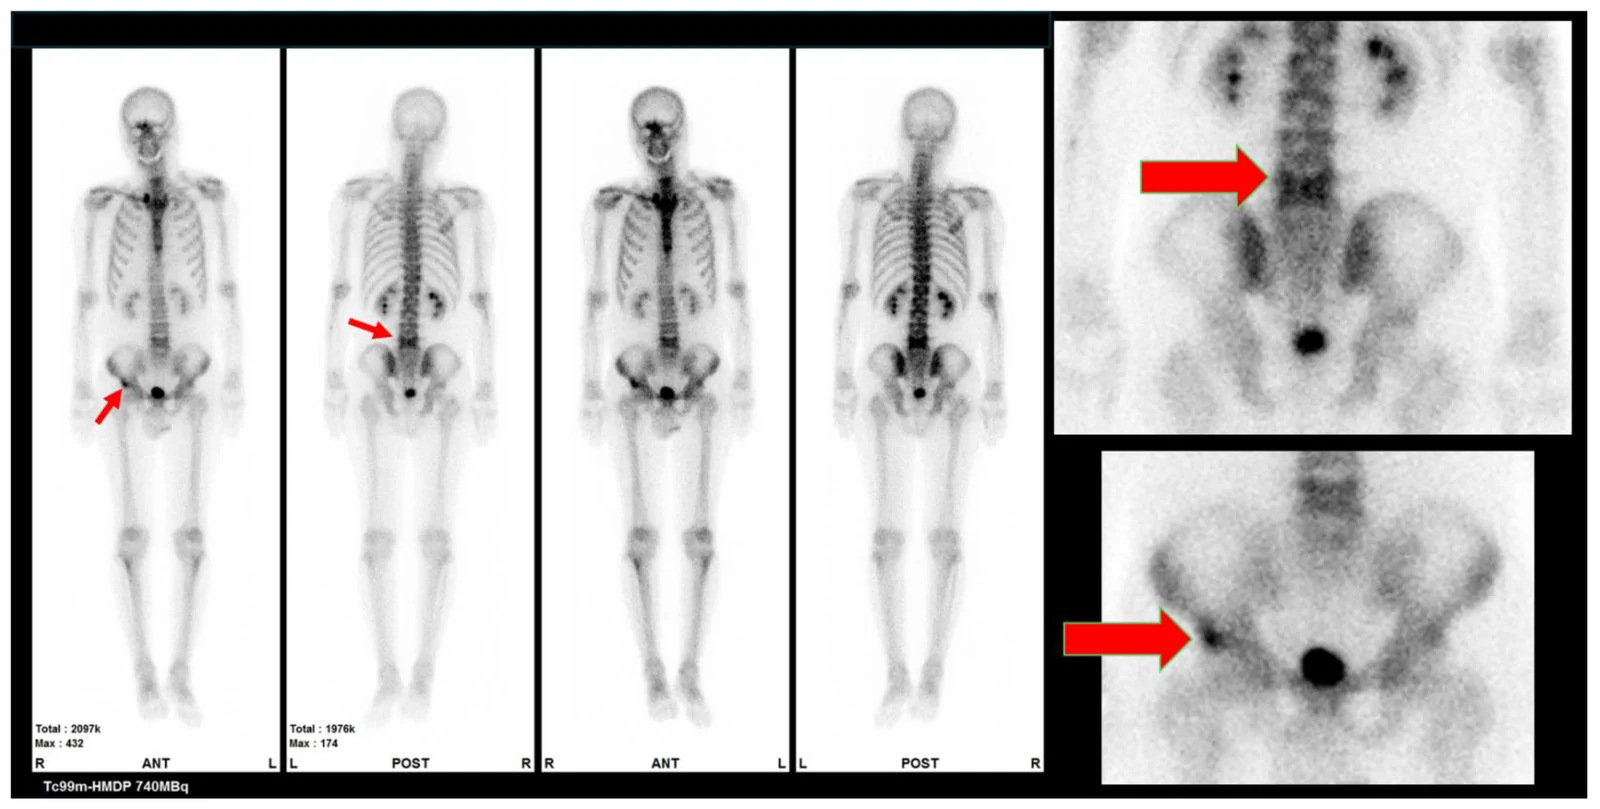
^99m^Tc-HMDP bone scintigraphy. Increased uptake suggestive of Paget disease of bone is observed in the pelvis and vertebral bodies (red arrows).
